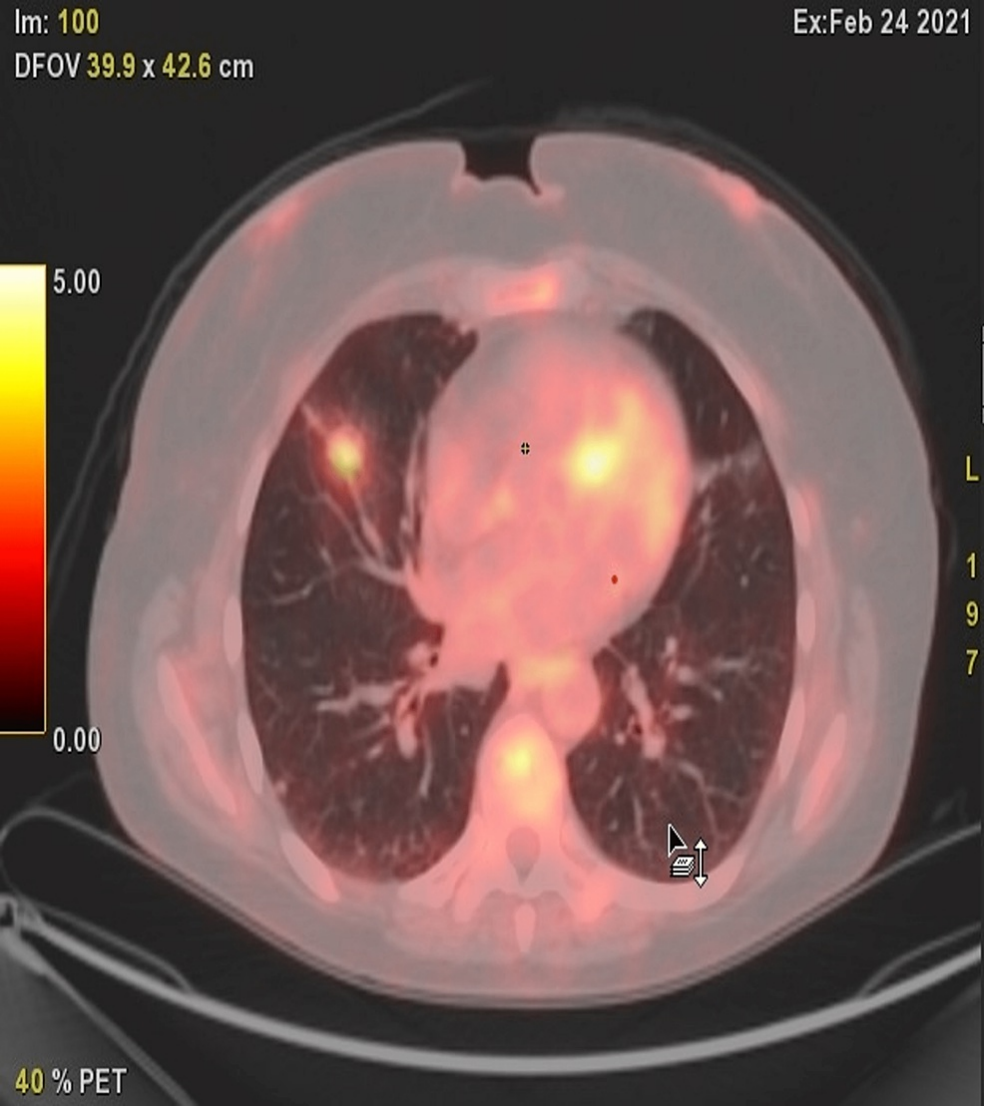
Chest PET-CT findings of a 56-year-old female patient: axial PET-CT section through lung base 1 cm diameter right middle lobe hypermetabolic nodule
